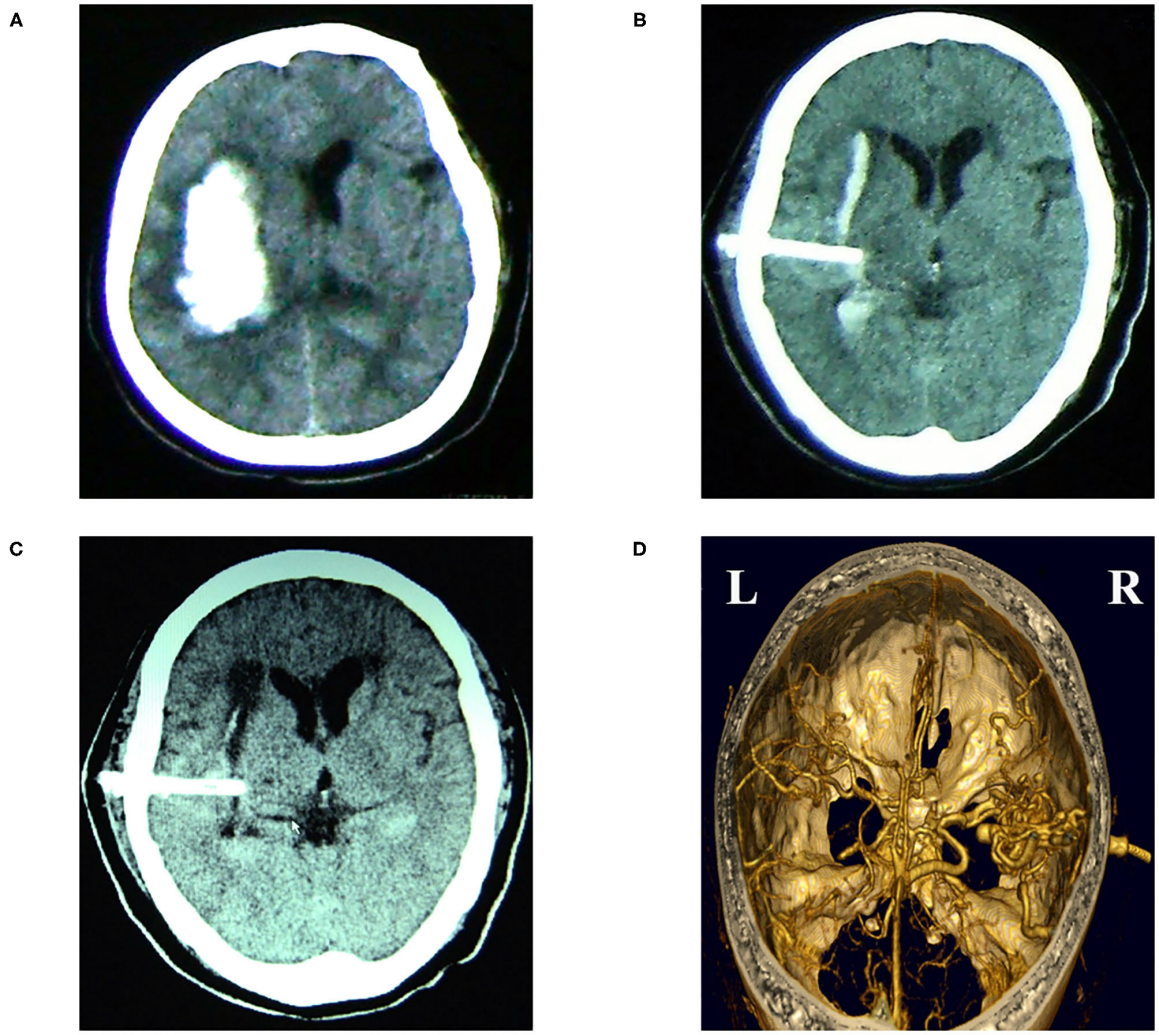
Images from a 65-year-old patient with the hypertensive right temporal hemorrhage who received cubic oriented stereotactic aspiration. (A) Preoperative CT image of the head. (B) A post-operative CT image of the head was carried out immediately, which showed that the soft drainage tube was inserted into the hematoma and the volume of the hematoma was reduced after aspiration. (C) CT image at 4 days after operation displayed that the hematoma was almost completely evacuated. (D) The post-operative CT angiography image of the head showed that the soft tube did not lead to the damage of vessels.
